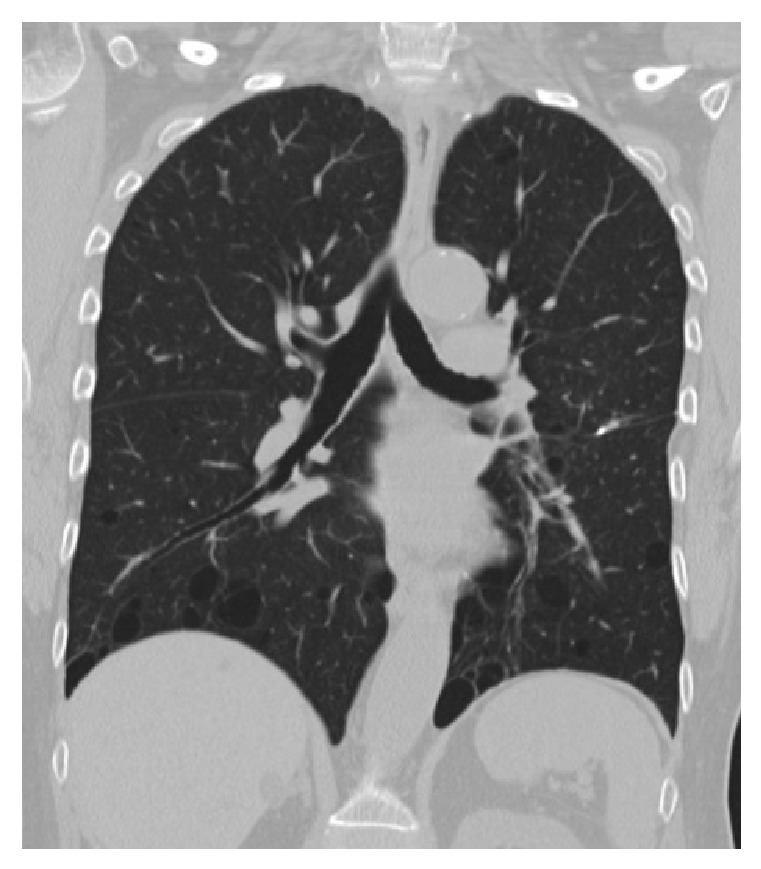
Coronal section of chest CT scan demonstrating numerous pulmonary cysts preferentially located in the lower lobes.
